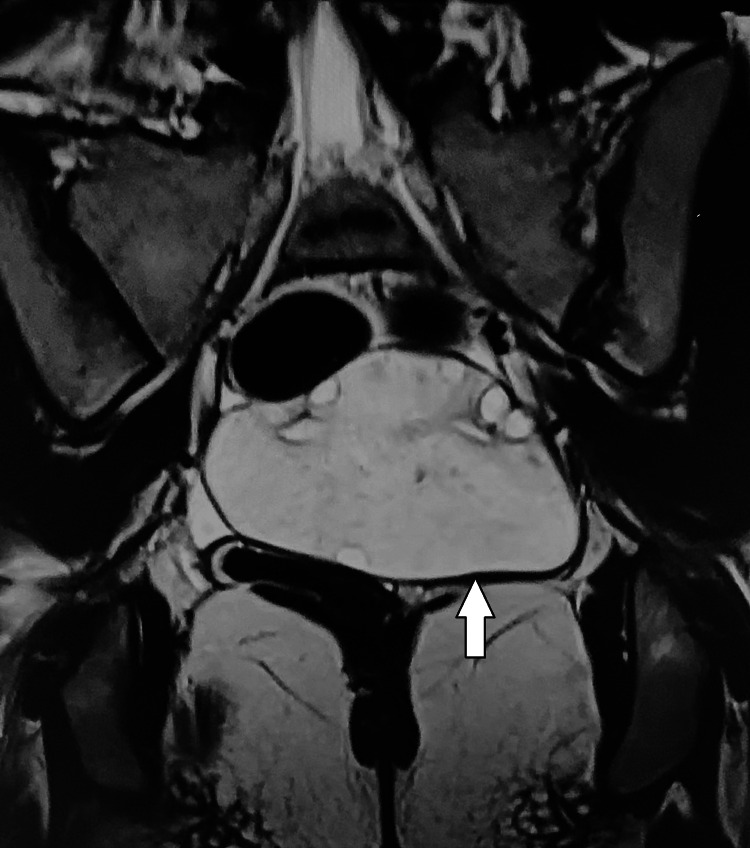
Coronal T2-weighted pelvic MRI image showing homogeneously high signal intensity within the enlarged left ovary (white arrow).
